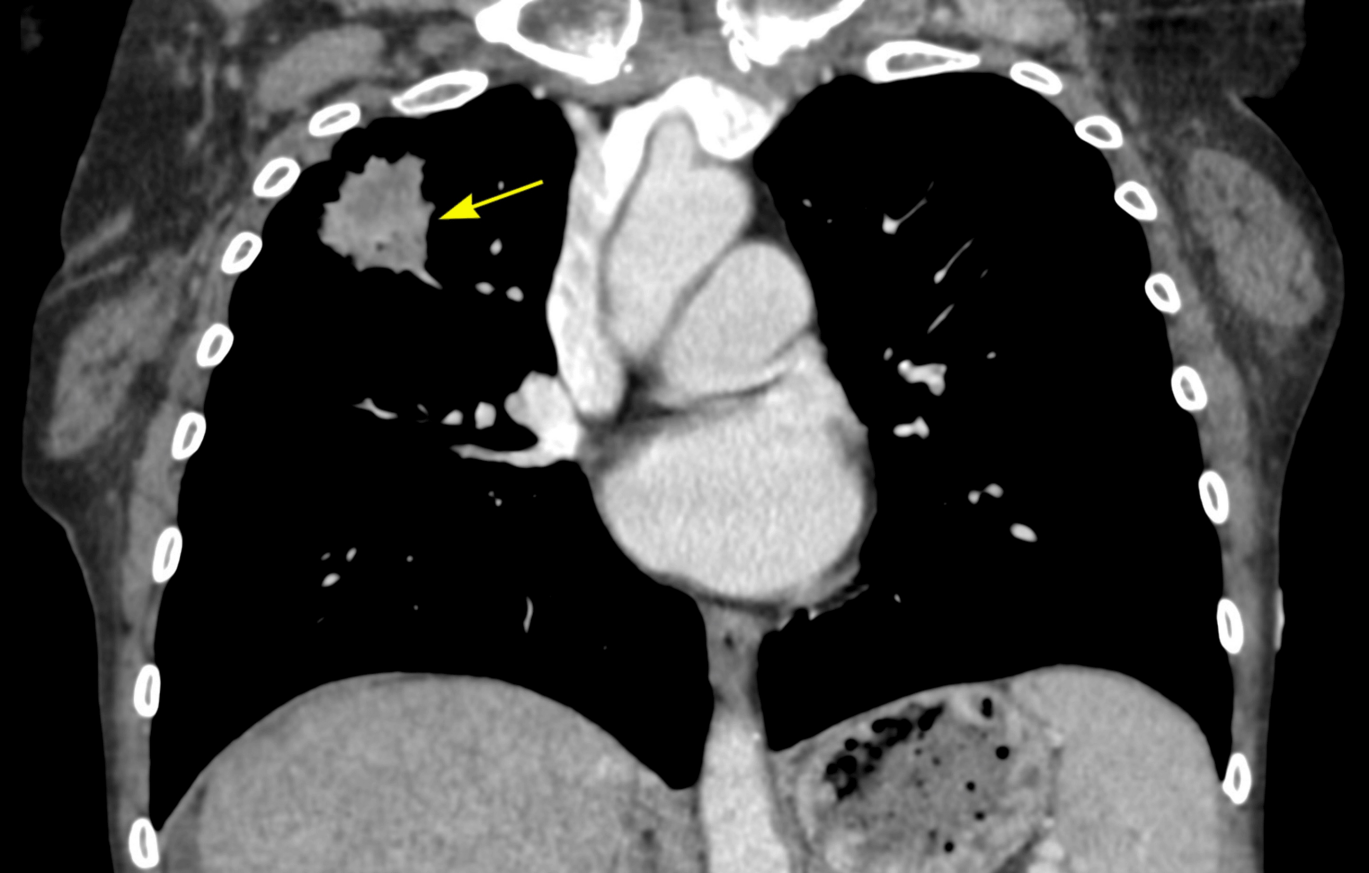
Chest CT (coronal) with contrast showing the 4-cm right upper lobe mass, compatible with malignancy (yellow arrow)
